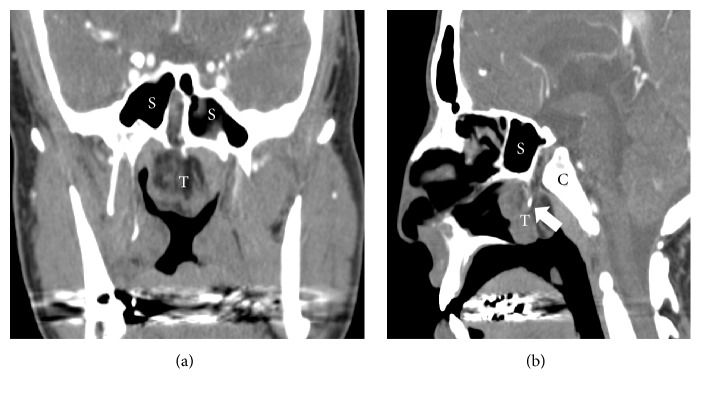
CT angiogram (CTA) imaging of pedunculated skull base lesion. (a) Coronal CT slice at level of the bilateral sphenoid sinuses (S), with tumor pedicle arising between sinus cavities, at typical site of intersinus septum. The 4 × 4 cm tumor (T) is composed of heterogeneously dense material, with suspected low-density (dark) fat seen. (b) CTA in sagittal view, showing relationship of tumor pedicle extending between anterior sphenoid sinus (S) and posterior clivus (C). Arrow shows the diminutive artery coursing through the pedicle, without direct communication with the ICA and vertebrobasilar system (not shown).
